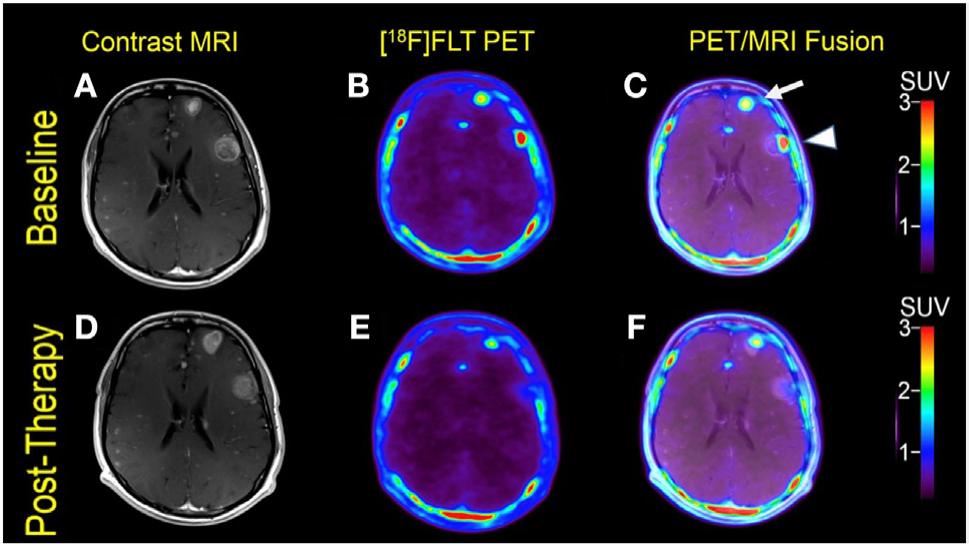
Case #2. Baseline magnetic resonance imaging (MRI) (A), fluorothymidine (FLT)–positron-emission tomography (PET) (B), and fused PET/MRI (C); follow-up MRI (D), FLT–PET (E), and fused PET/MRI (F) at 1 month. Among multiple melanoma brain metastases, the left anterior frontal lobe lesion (arrow) showed a slight interval increase in FLT uptake and size [SUV ratio (SUVR) from 12.6 to 13.5; size from 1.7 to 2.0 cm]; the left lateral frontal lobe lesion (arrowhead) demonstrated interval decrease in FLT uptake from SUVR of 11.3–6.3, while the size remained stable at 2.3 cm. Same T1-weighted MRI parameters as in Figure 1.
